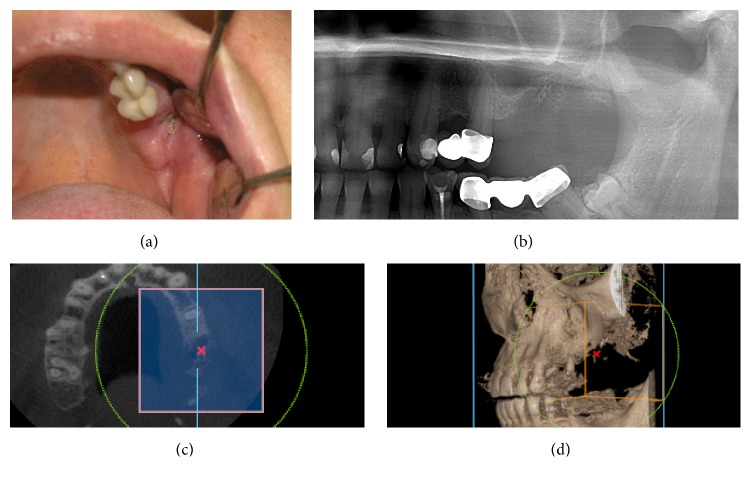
Stage I MRONJ. (a) Intraoral detail. (b) Orthopantomograph and CBCT 3D mode and cross section analysis excluded any bony sequestrum. (c) Axial view of CBCT confirmed our findings. (d) 3D mode view in Scanora 3D software is especially useful tool. Using 3D zoom tool, it is very easy to eliminate other structures which could interfere with region of interest.
